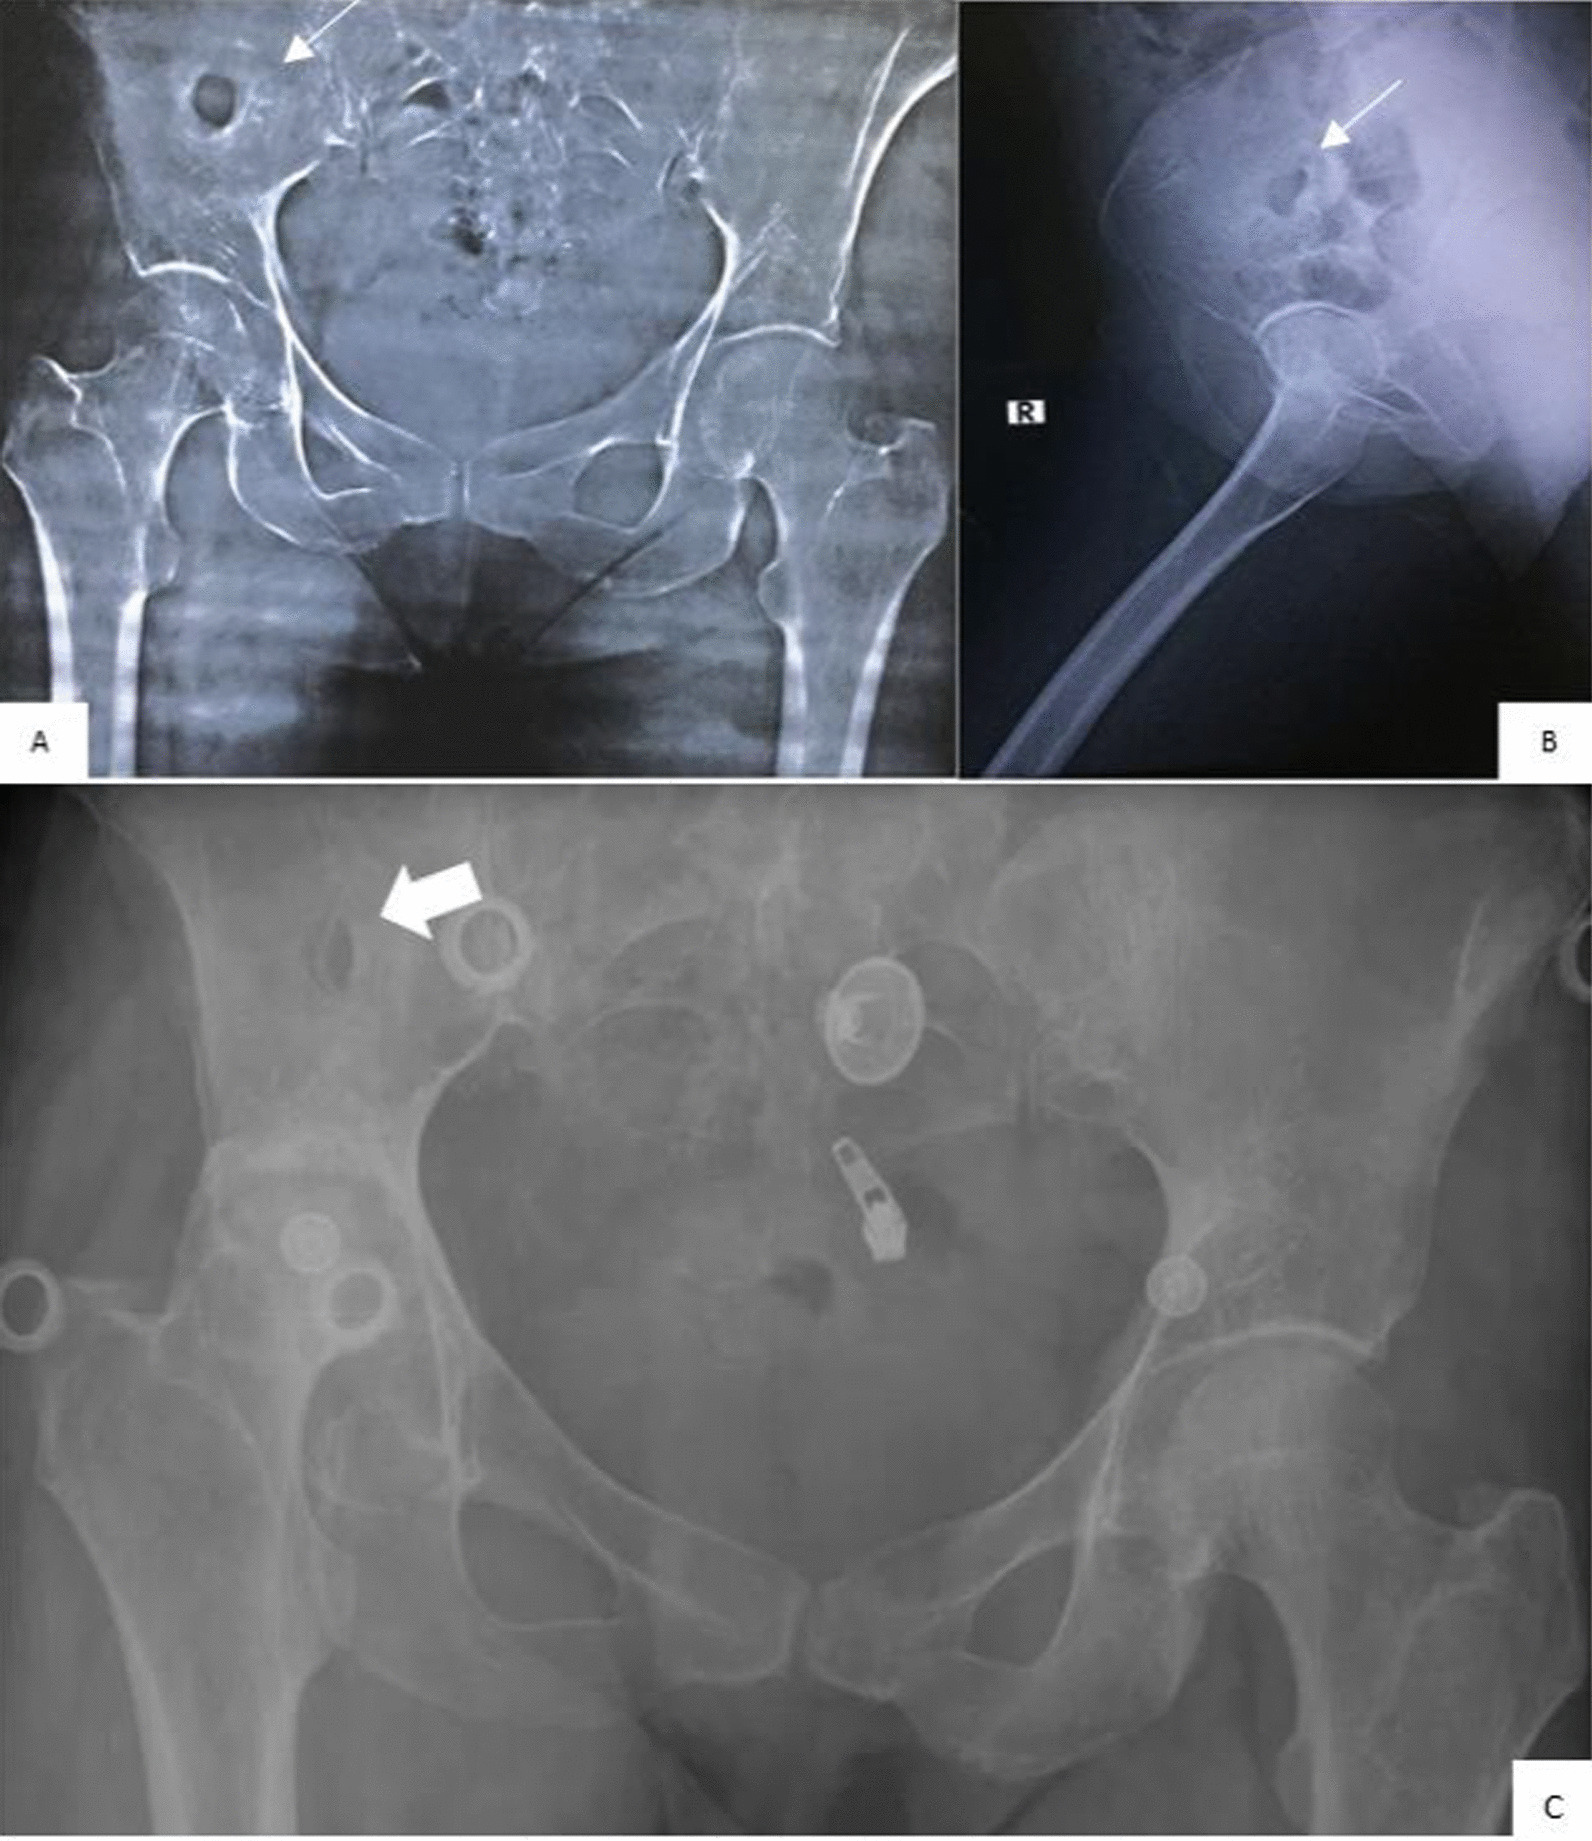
Posteroanterior (A) and side view (B) of the pelvic, showing necrosis in the right iliac wing (white arrow). C Posttreatment follow-up X-ray showing the remaining necrosis in the right ileum wing (white arrow)
